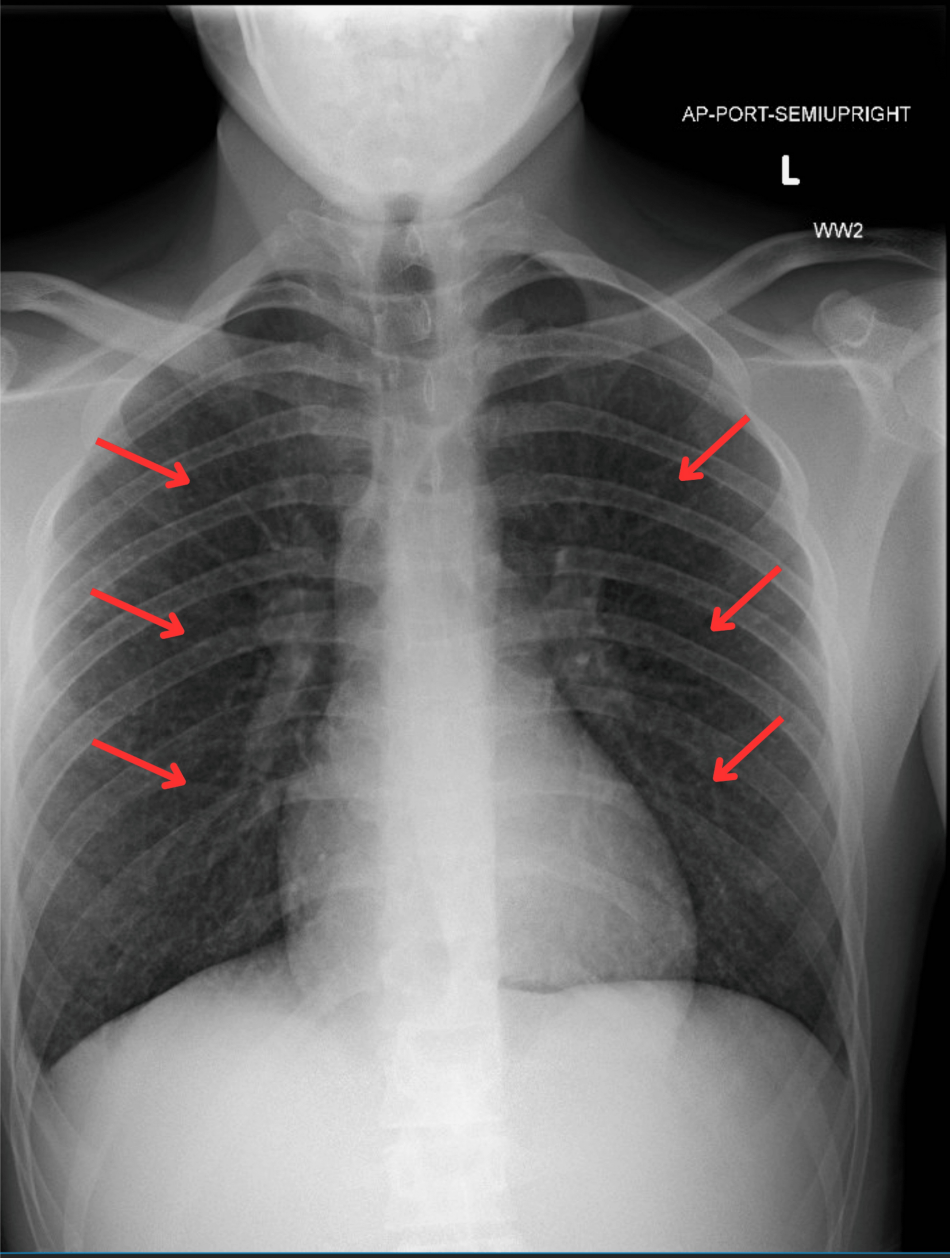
Chest X-ray demonstrating reticular opacities (red arrows) in bilateral lung fields
